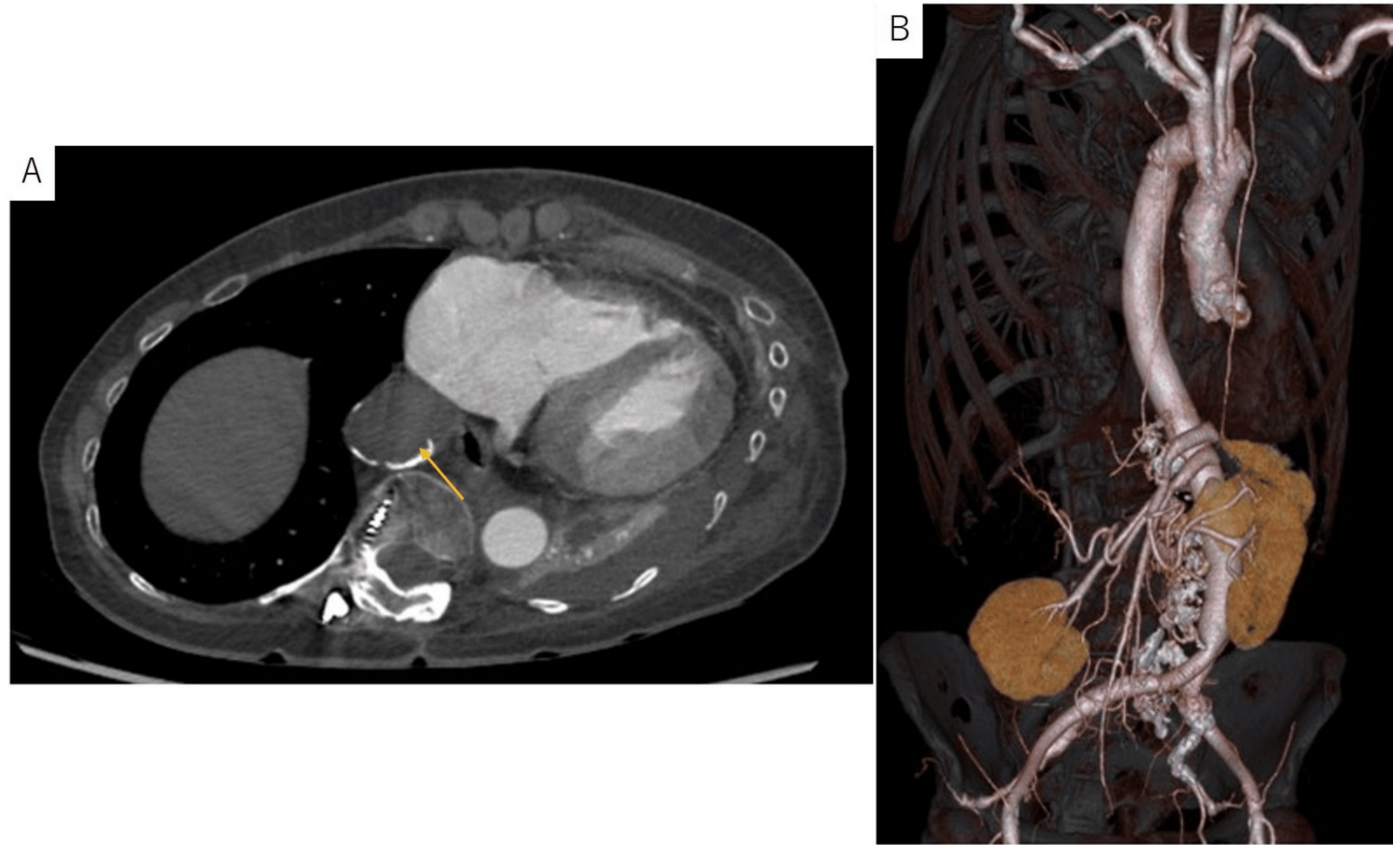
Patient’s postoperative CT findings (A) The excluded thoracic aneurysm was completely thrombosed without any intercostal inflow(arrow). (B) Three-dimensional CT image.
